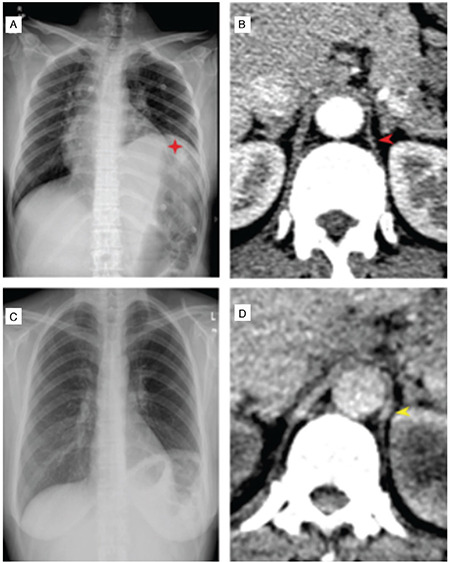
A) Elevated hemidiaphragm and loss of volume on the left lung with normal bilateral lung parenchyma on chest X-ray. B) Thorax axial CT image showing the thinning of the diaphragmatic crura (red arrowhead) at the time of presentation. C) The improvement in the lung volume and diaphragm and D) diaphragmatic crura (yellow arrowhead) after immunosuppressive treatment.
